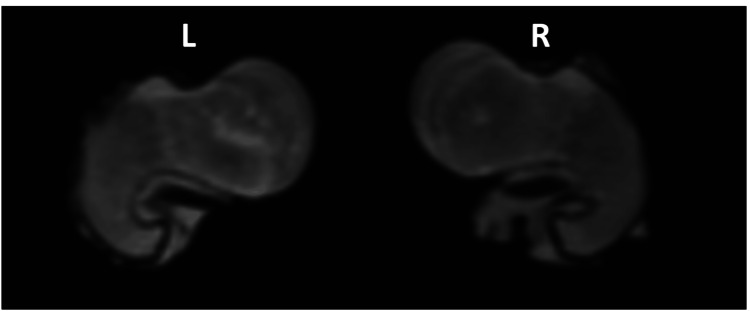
MRI image of the femoral neck in the operated and non-operated femur with both femoral condyles aligned parallel to the scanning table showing the difference in femoral anteversion: L, left operated femur; R, right non-operated femur.
